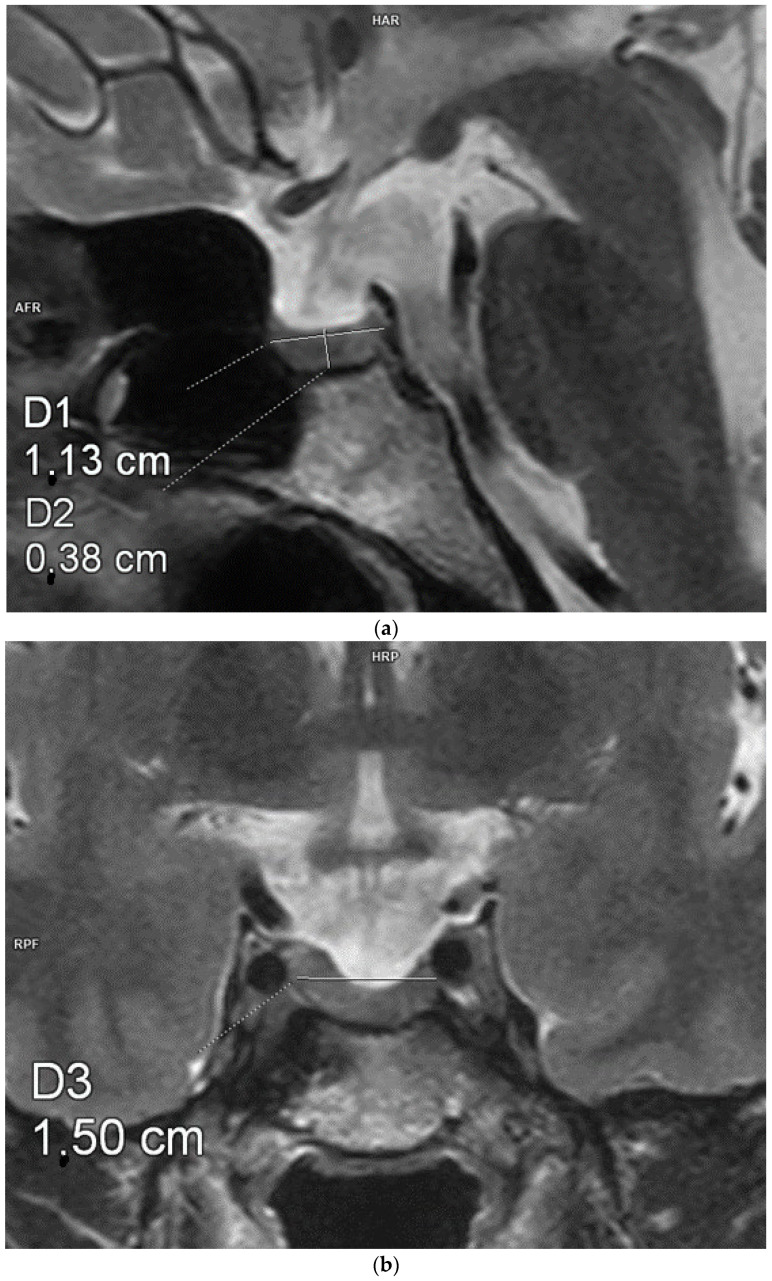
Measurements of the pituitary gland. Magnetic resonance imaging, T2-weighted images in the sagittal (a) and coronal (b) planes. The craniocaudal, transverse, and anterioposterior dimensions of the pituitary gland are 3.8 mm × 11.3 mm × 15 mm.
